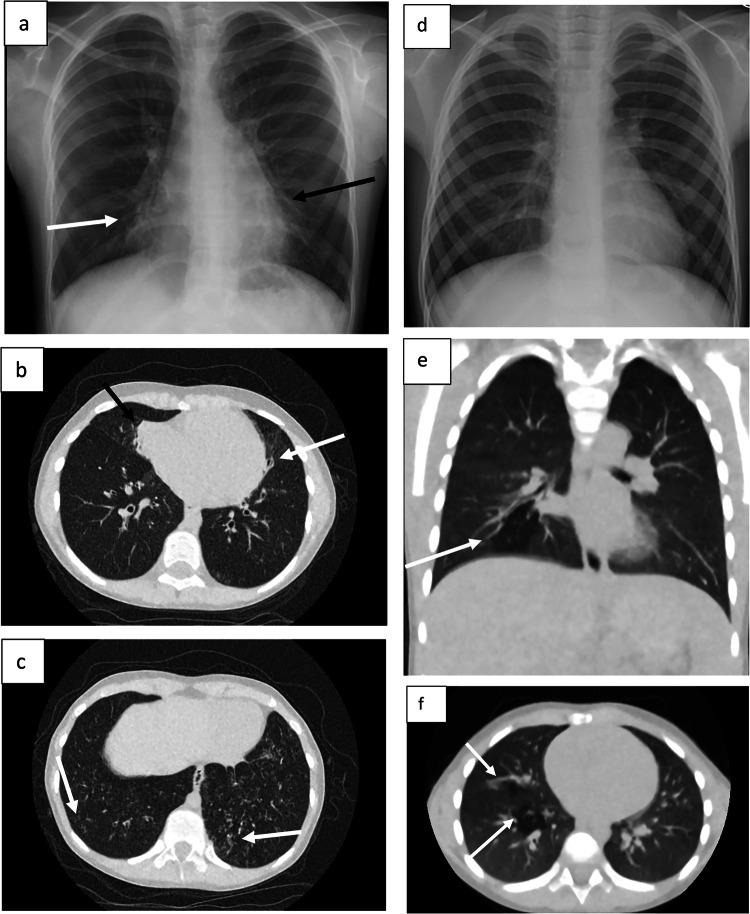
a–c Plain radiographic and CT features of a 7-year-old with CF. a Chest radiograph shows over-inflated lungs with bronchial wall thickening and mild dilatation in the middle lobe (white arrow) and lingula (black arrow). b ULDCT shows volume loss in the right middle lobe with subsegmental atelectasis (black arrow) in the medial segment. There is mild bronchiectasis in the lingula (white arrow) and in both lower lobes. c ULDCT shows diffuse bronchial wall thickening, mild bronchiectasis and subtle tree-in-bud opacification bi-basally (white arrows). d–f Plain radiographic and CT features of 5-year-old with CF. d Chest radiograph shows no significant abnormalities. e ULDCT coronal image showing a focal area of regional hypoattenuation (white arrow) in the right lower lobe, giving a “patchwork appearance”; findings are consistent with mosaic attenuation and suggest air-trapping and small airways disease. f ULDCT axial image showing additional areas of mosaic attenuation (white arrow), again suggesting air trapping and diffuse small airways disease. There is also linear opacification in the right middle lobe (white arrow), consistent with plate atelectasis
